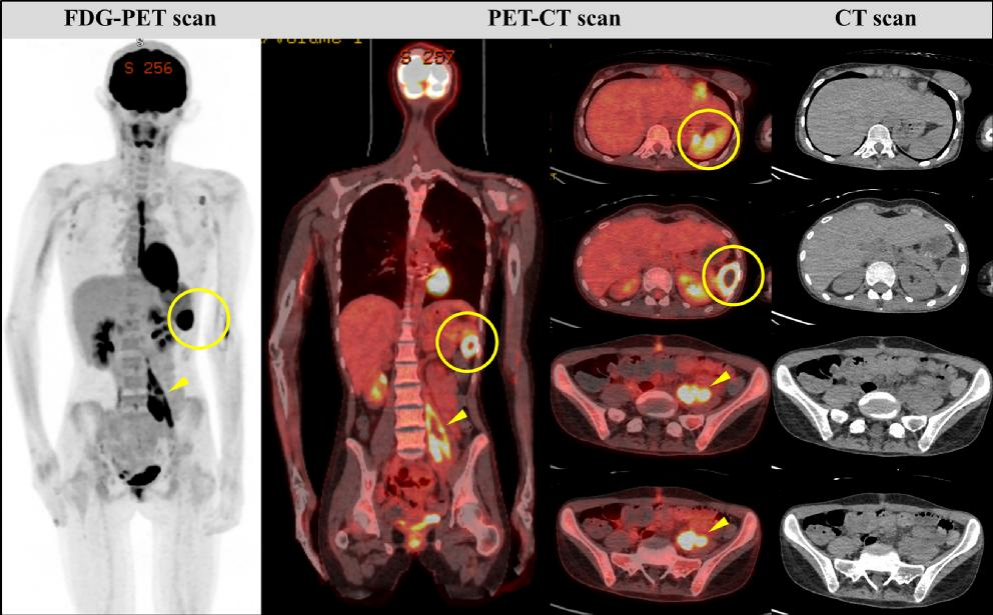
Whole-body PET-CT imaging reveals increased FDG uptake in the spleen (yellow ring) and left psoas muscle (yellow arrowhead). In the context of prior splenic infarction and abscess, and rupture of a left internal iliac pseudoaneurysm, these findings indicate ongoing splenic abscess and infected haematoma within the left psoas muscle. FDG, 18F-fluorodeoxyglucose; PET, positron emission tomography; CT, computed tomography.
